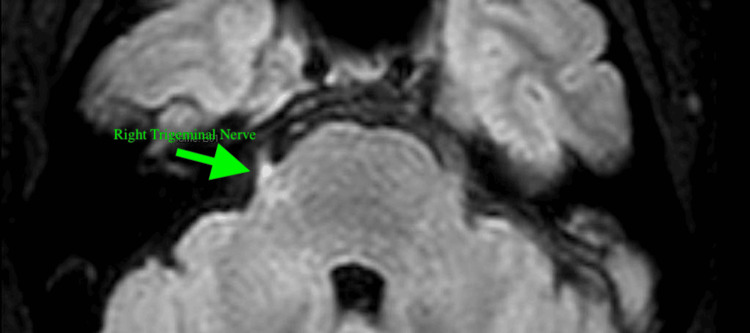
MRI brain evaluating right trigeminal nerve Green arrow: Symmetric T2 hyperintense signal at the root entry zone and cisternal segment of the right trigeminal nerve
